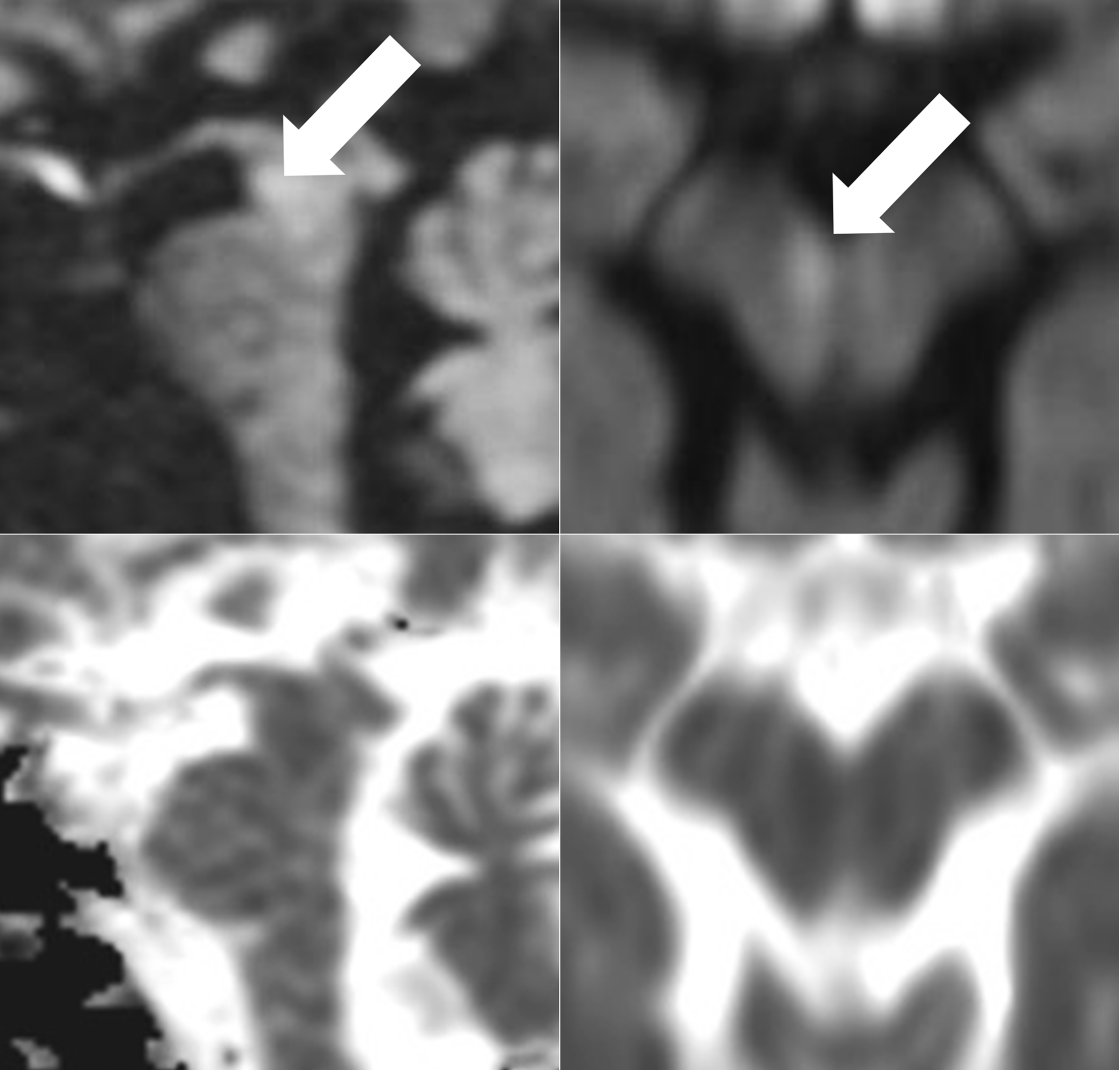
Lesion only detectable on review of both the axial and sagittal DWI together. B-1000 images and ADC-maps of the lesion (arrows) that was only detected on review both the axial (left) and the sagittal (right) DWI together (R_ax+sag).
